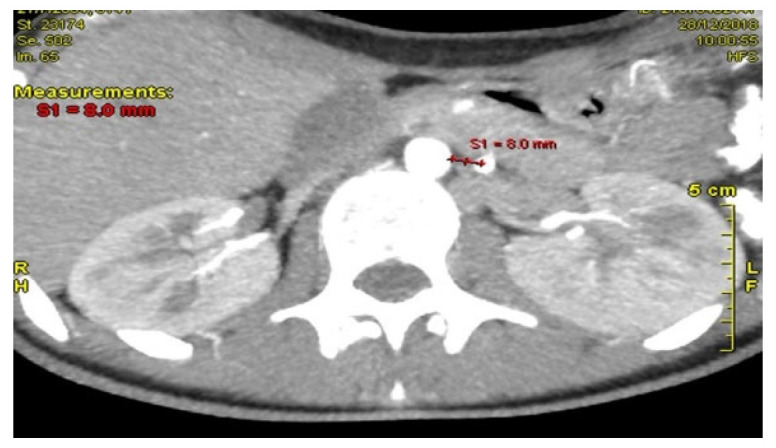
CTA: The aortomesenteric angle was significantly reduced to 6°.
